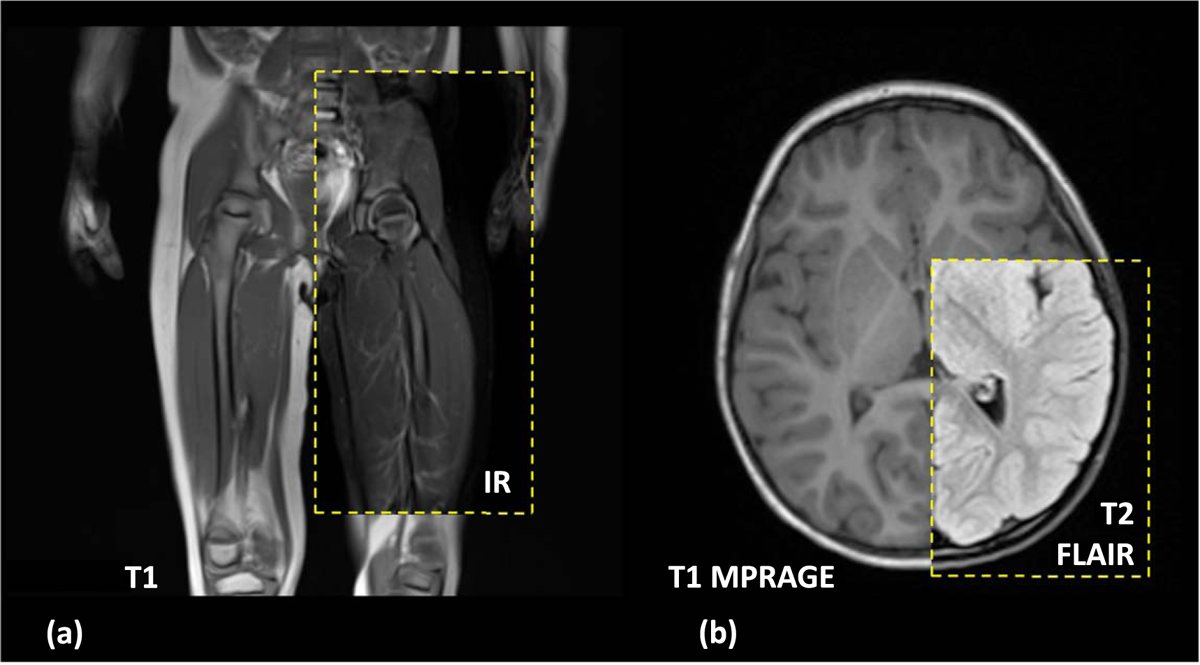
Registration of different MRI scans: (a) coronal view of T1 MRI registered with IR sequence, the contrast of vessels and intervertebral discs was enhanced in IR scans. (b) Axial view of T1 MRRAGE sequence registered with T2 Flair sequence where fat suppression is shown in the subcutaneous fat and the myelinated white matter tracts.
